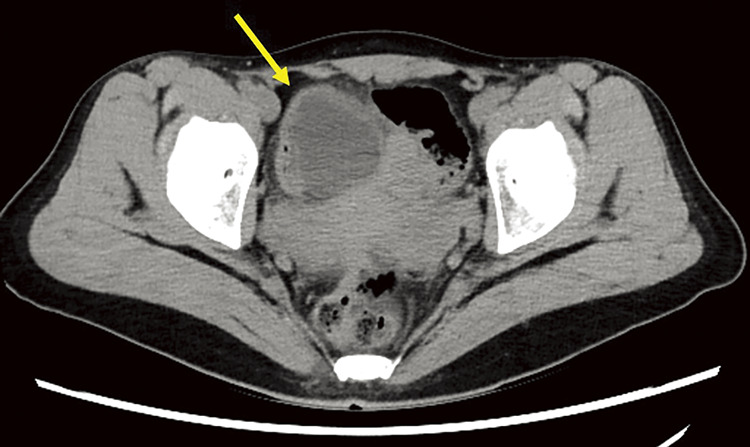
Computed tomography shows a cystic mass shadow on the right ventral side of the uterus (arrow).
